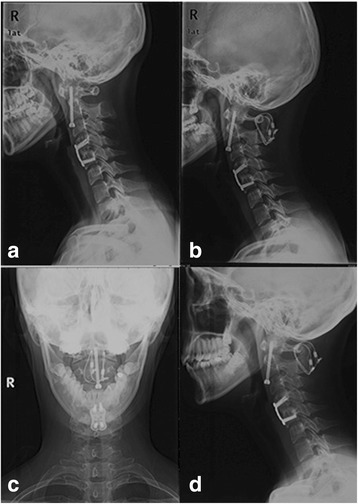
A 19-year-old woman had type II odontoid fracture caused by traffic accident. a Lateral radiograph showed atlantoaxial dislocation was still present. b Lateral radiograph obtained at 1 day after the Gallie fixation showed good reduction of atlas. c, d Open-mouth and lateral radiographs obtained at 18 months after the Gallie fixation, showing bone fusion
